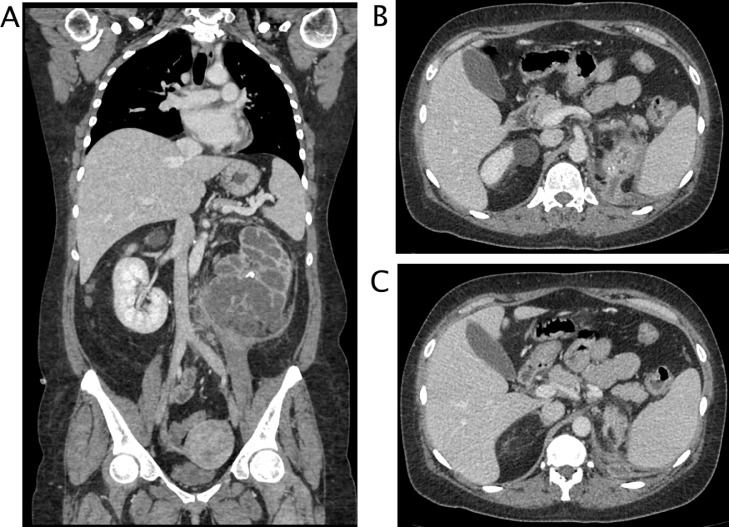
CT with intravenous contrast, parenchymal phase. (A) multiplanar coronal plane recontruction . The left kidney is enlarged, calico-pelvic system in markedly dilated with thickening of parietal wall and calyceal corticalization. Calculi are appreciable in the pelvis. Perirenal fascia is thickened and there is stranding of the perirenal fat. On the lower pole, fluid dilated calyces are not well demarcated and close to perirenal fascia. Iliopsoas muscle appears enlarged and edematous as inflamed secondarily by the infectious renal process. Nodes are detectable. (B, C) axial images. On the upper pole of the left kidney a fluid collection with hyperemic wall is appreciable, the collection goes through the diaphragm that appears focally thickened.
